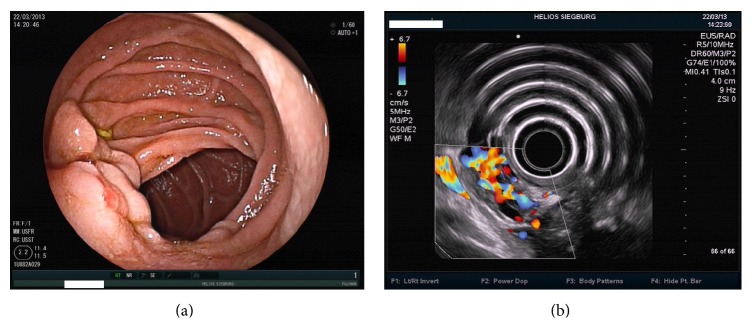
Duodenal varices in a patient with portal vein and splenic vein thrombosis: (a) endoscopic picture, (b) endosonographic picture.
